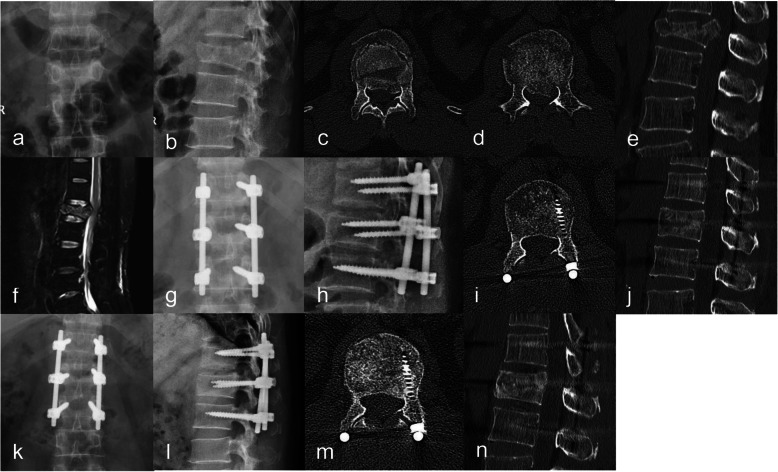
A 39-year-old man was diagnosed with an L1 vertebral burst fracture. a Preoperative anterior and posterior X-ray of the lumbar spine. b Preoperative lateral X-ray of the lumbar spine. c Preoperative CT axial position. d Preoperative CT axial position. e Preoperative CT sagittal position. f Preoperative MRI sagittal position. g Anterior and posterior X-ray of the lumbar spine on 3 days after the operation. h Lateral X-ray of the lumbar spine on 3 days after the operation. i CT axial position on 3 days after the operation. j CT sagittal position on 3 days after the operation. k Anterior and posterior X-ray of the lumbar spine on the last follow-up. l Lateral X-ray of the lumbar spine on the last follow-up. m CT axial position on the last follow-up. n CT sagittal position on the last follow-up
